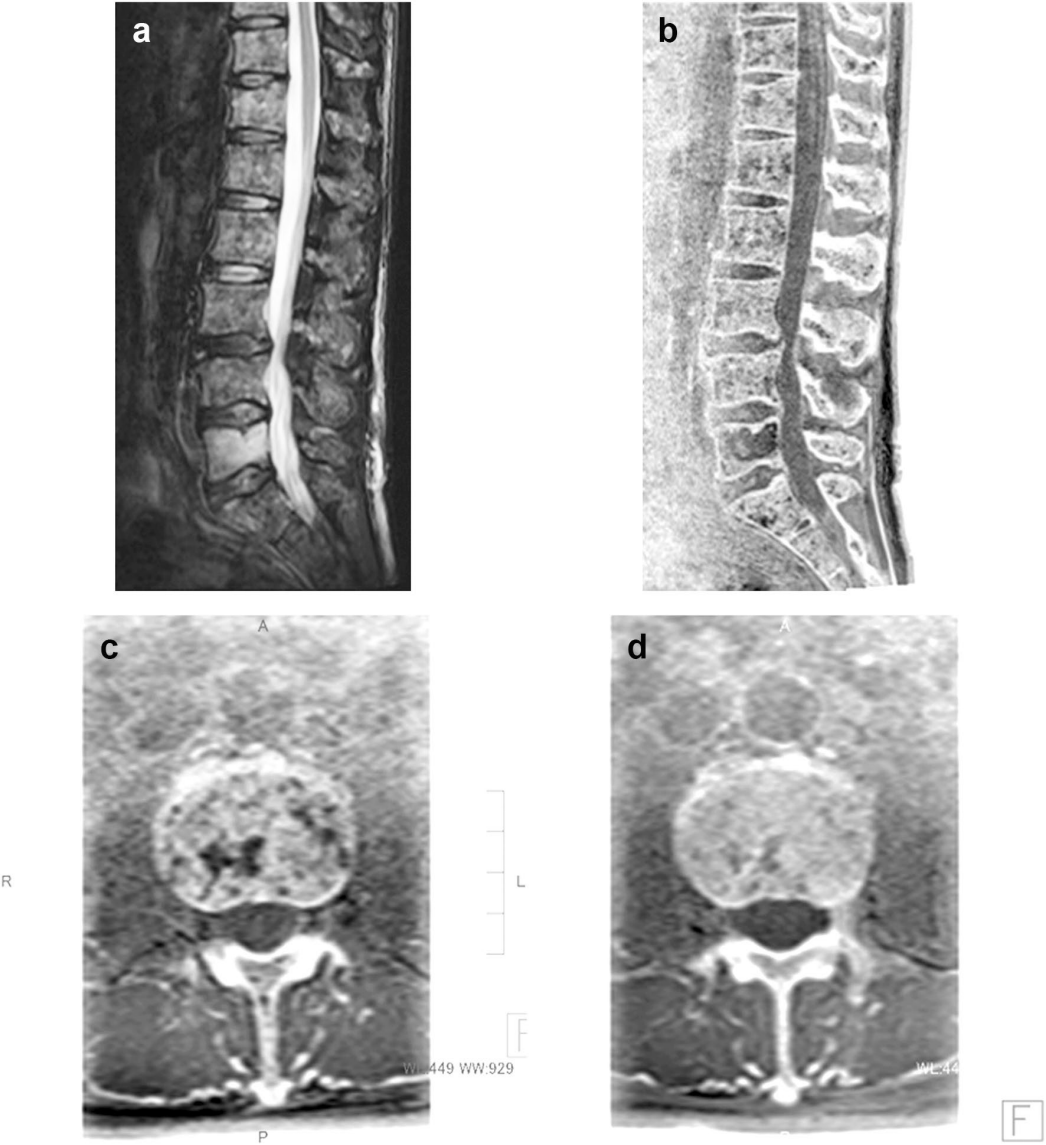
Plasma cell myeloma. Spinal metastasis is one of the common complications of plasma cell myeloma and often develops multiple destructive lesions. Sagittal fat-suppressed T2-weighted MR image shows numerous hyperintense lesions in the lumbar vertebrae and sacrum in a 60-year-old male patient (a). A mild compression fracture of the L5 body is also noted. Sagittal and axial MR bone images (b) and (c) show the lesions more clearly. Axial MR bone image (d) obtained at the same level after 3.5 months of chemotherapy shows the resolution of the lesions
